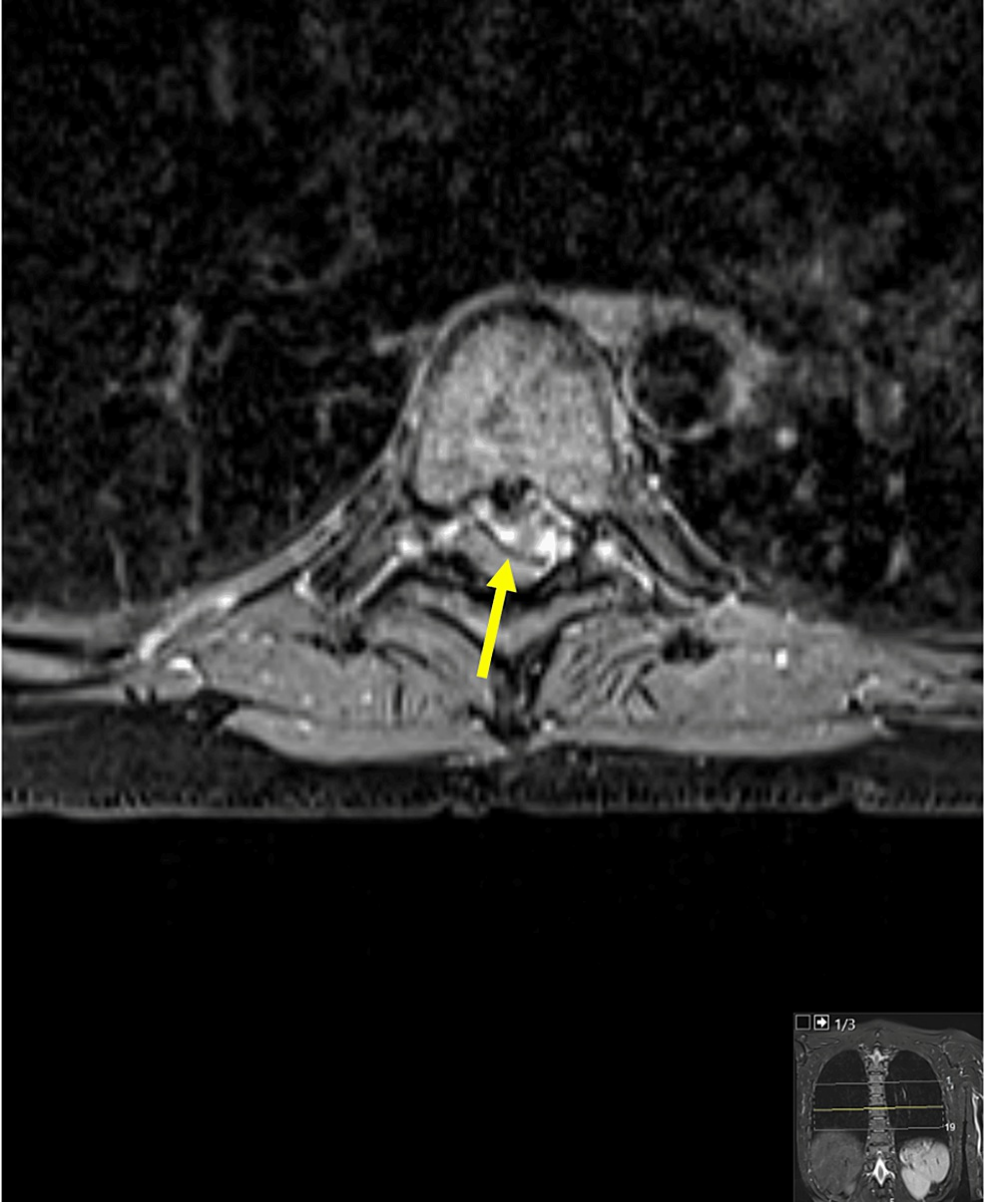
MRI transverse image (T7) showing compression of the spinal cord. Arrow: herniated lesion compressing the spinal cord.
